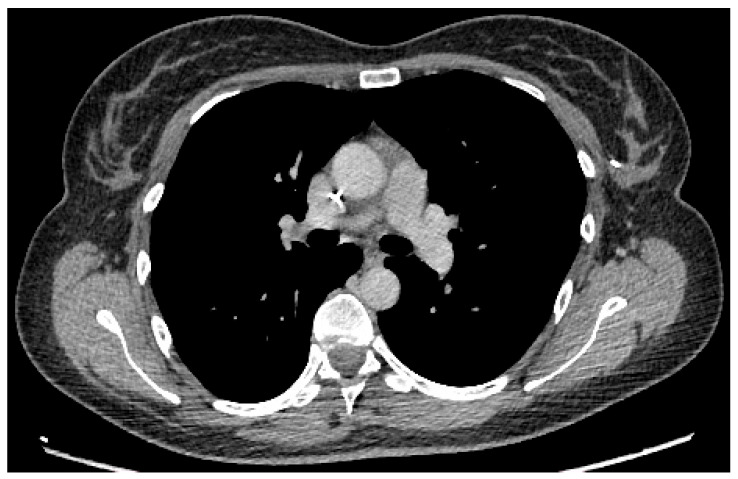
Two months of therapy CT scan control (institutional patient data).
